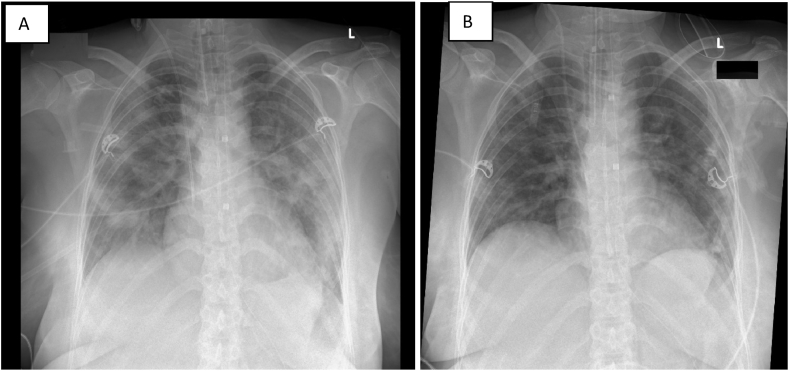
Chest x-rays: A: on 7.1.2022: after orotracheal intubation; B: on 16.1.2022 before extubation.
